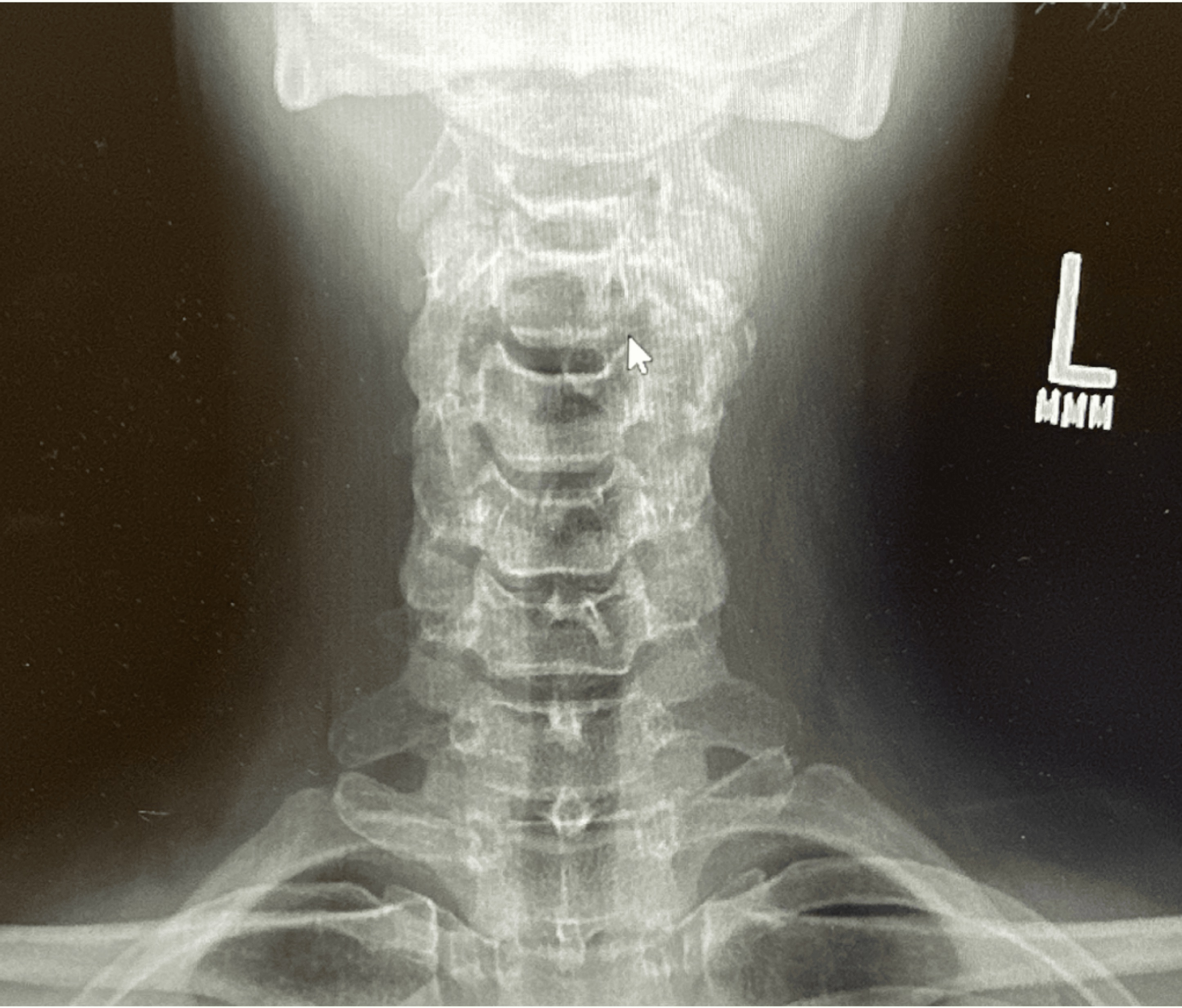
Posterior cervical X-Ray showing degenerative changes at C5–C6 with reversal of cervical lordosis L: Left
